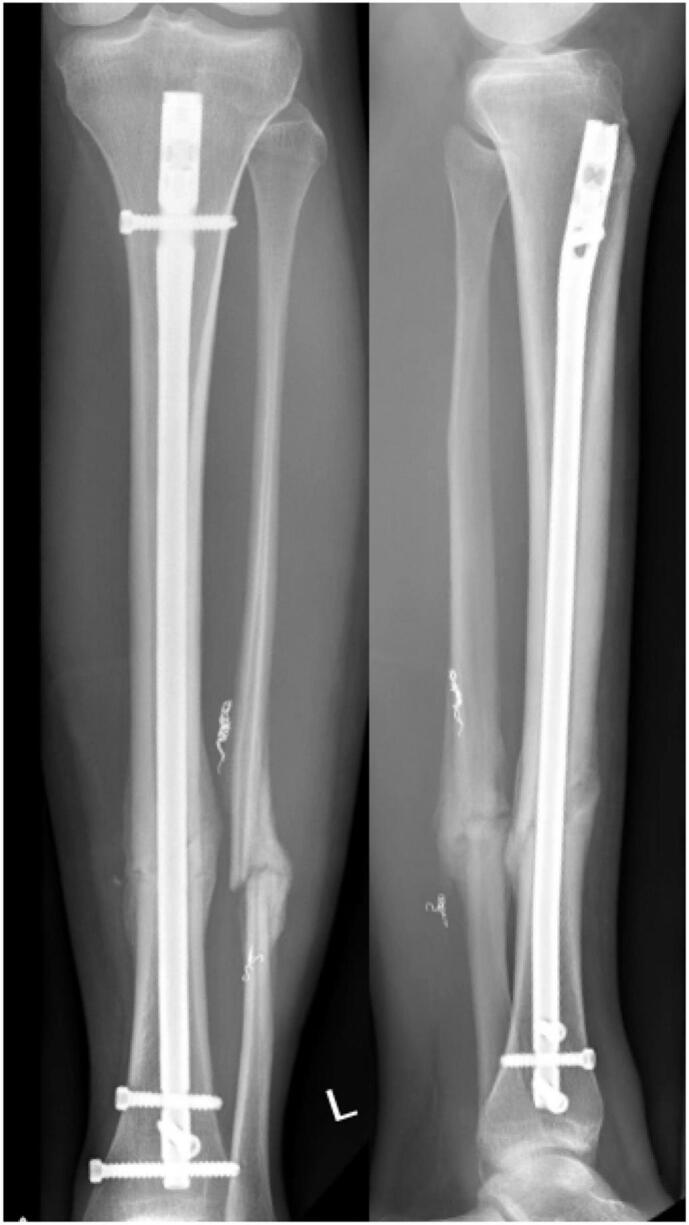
Postoperative X-ray control 6 weeks following surgery.
